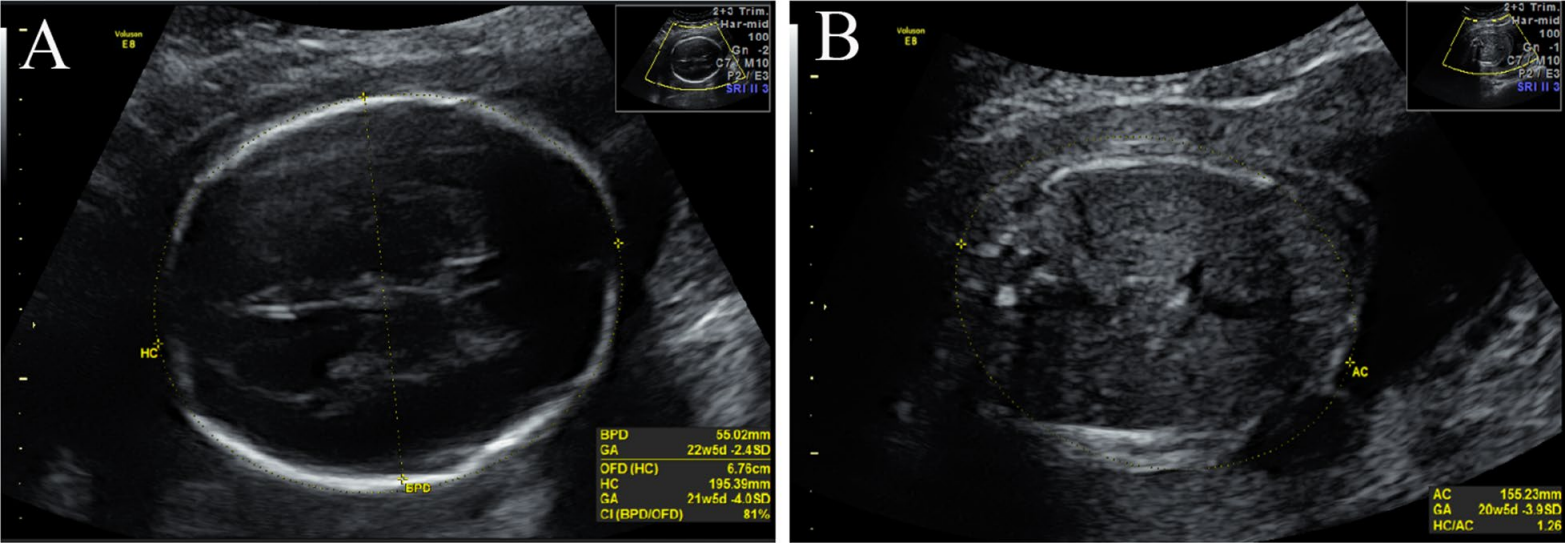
Fetal ultrasound at 25 weeks of gestation indicates severe growth restriction. BPD, HC (A) and AC (B) measure below the 3rd percentile
